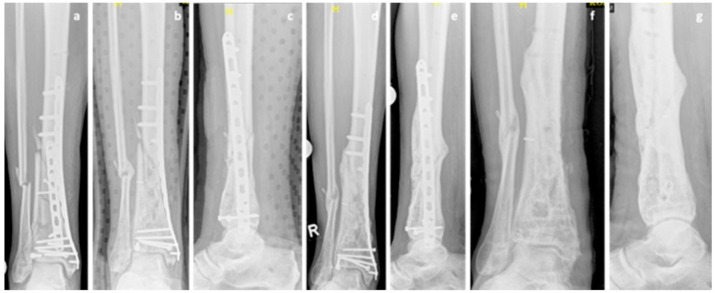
Case 5, x-rays showing the postoperative outcome after initial treatment of grade 1 open tibial and fibular fracture (a), after partial hardware removal (anterior tibial plate) and autologous cancellous bone grafting at the presence of an atrophic tibia nonunion 9 months after trauma (b,c) and after achieving bone union 18 months after the initial injury (d,e). Final result after hardware removal 24 months after trauma (f,g).
